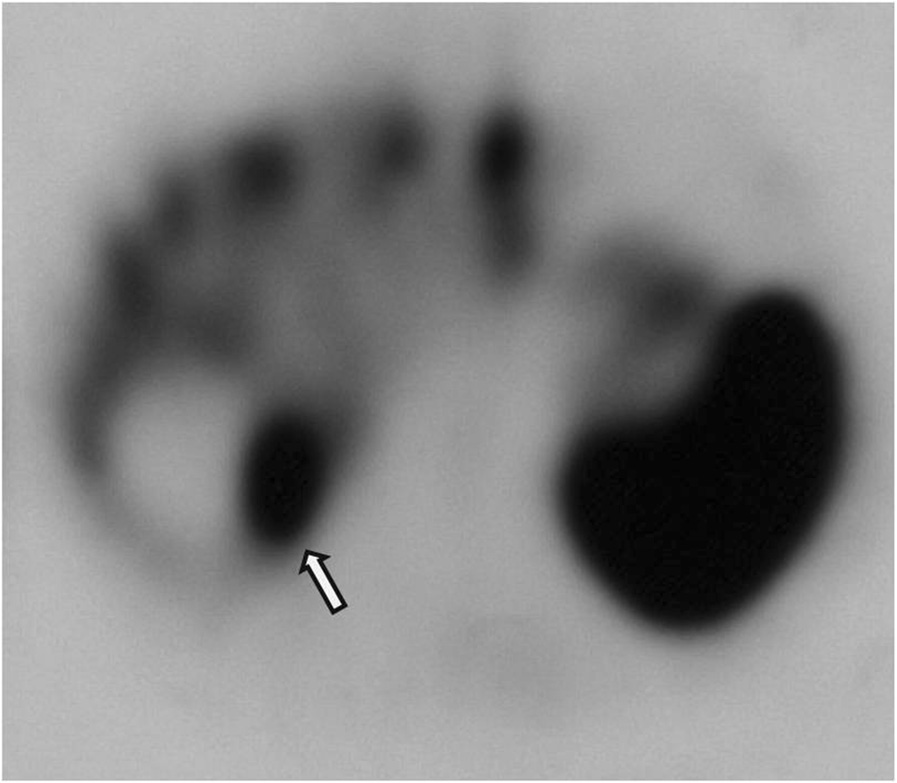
^111^Indium-pentetreotide scintigraphy showing multiple intrahepatic hypercaptations; the more posterior of them (arrow) is near to a large cyst sited in segment VII.
